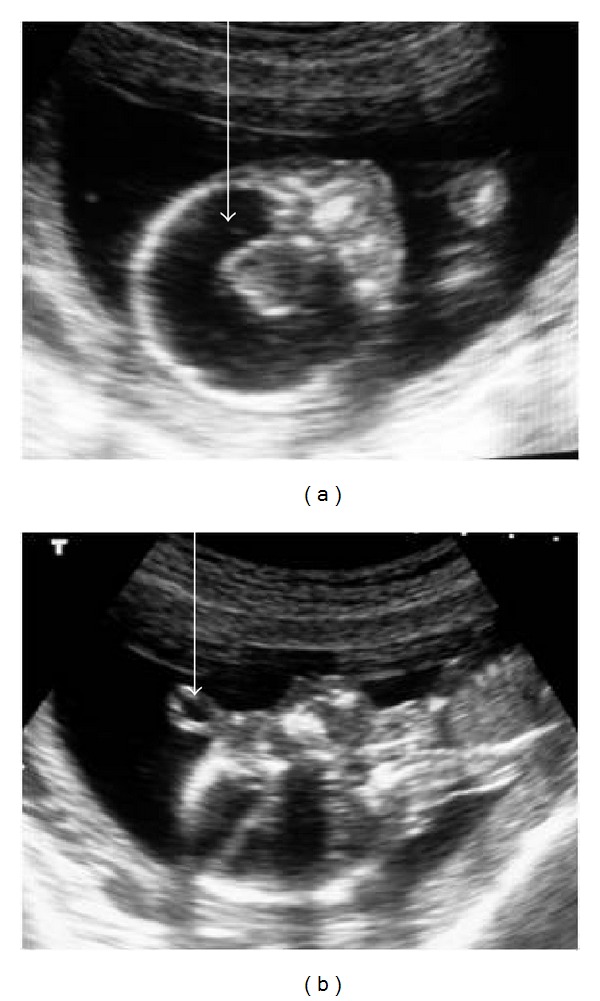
(a) Coronal ultrasound image showing the fused thalami in the centre and large monoventricle (thick white arrow). The amniotic fluid around the fetus is normal in quantity. (b) Sagittal ultrasound image showing the proboscis (thin white arrow) as a tubular cystic projection in the frontal region.
